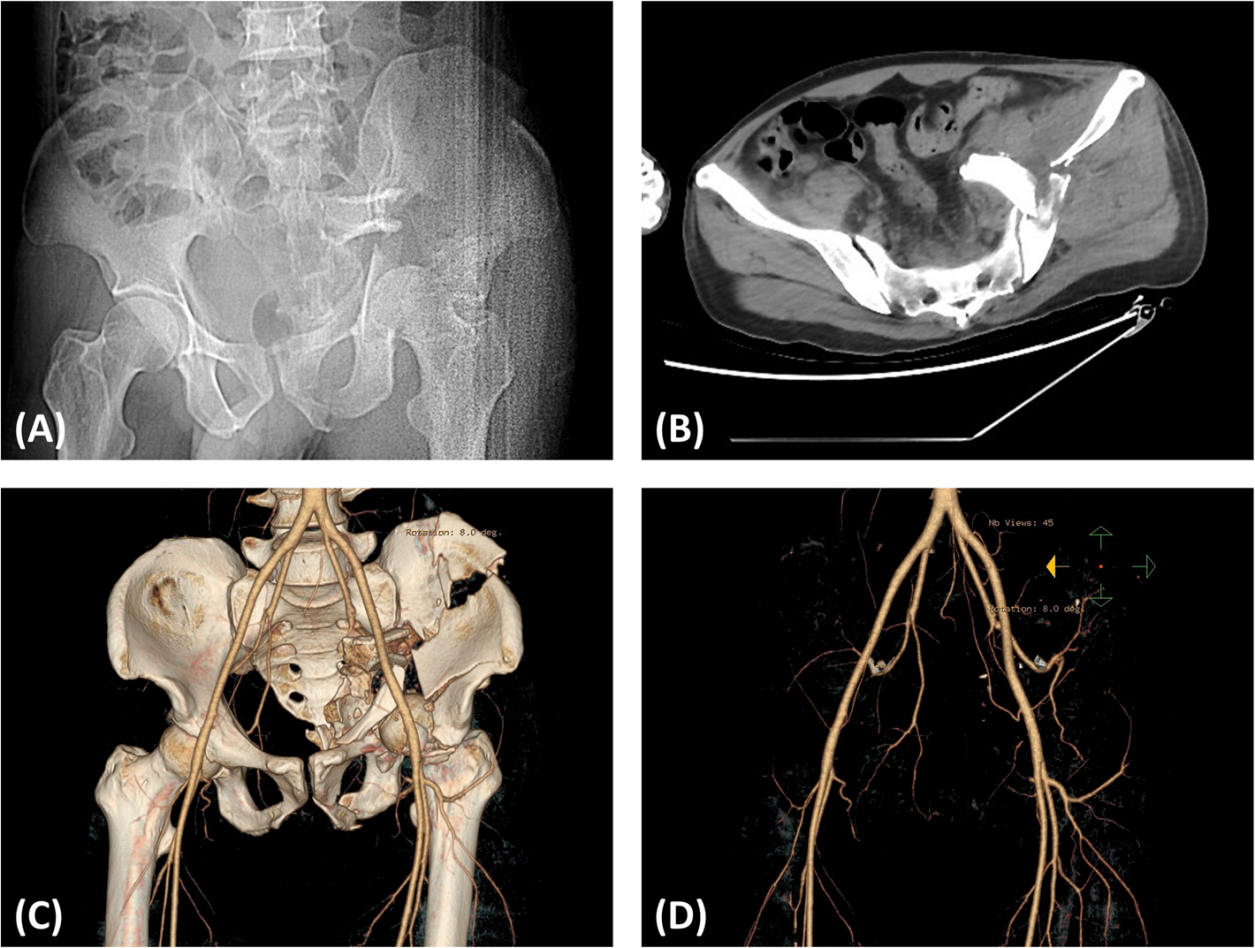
A patient (age between 40 and 50) sustained a fracture of his left acetabulum after a fall. Due to thoracic and head trauma, the surgery was performed 67 days later when the patient was in stable general condition. a Preoperative anteroposterior radiographs and (b) computed tomography scans of the pelvis were performed. Ruptured and rotated fragments of the sciatic notch were suspected to be causing injury to the superior gluteal artery. c To obtain a clear diagnosis, the patient was examined with computed tomography angiography. d No obvious arterial damage was found on computed tomography angiography; however, geometric information regarding fractures and arteries cannot be obtained from two-dimensional images
